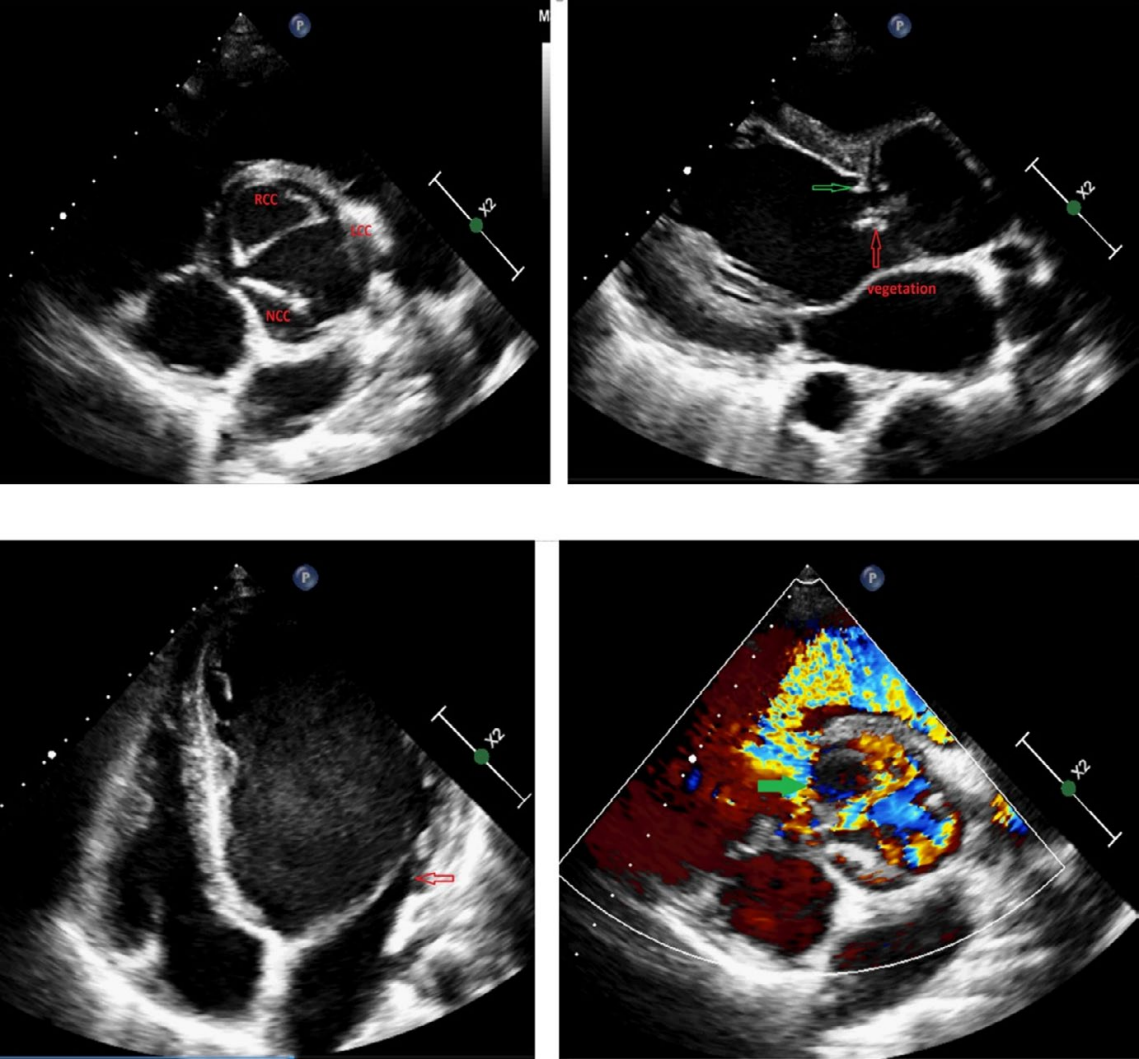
Top left: parasternal short‐axis view of aortic valve that is tricuspid with dissected RCC, top right: parasternal long‐axis view that showed small subaortic web (green arrow) and also vegetation on aortic valve (red arrow), bottom left: 4 chamber apical view showed left ventricular enlargement with severe hypoplasia of PMVL (red arrow), bottom right: parasternal short‐axis view of small perimembranous VSD (green arrow) with left to right shunt. RCC: right coronary cusp, LCC: left coronary cusp, NCC: noncoronary cusp, PMVL: posterior mitral valve leaflet, VSD: ventricular septal defect
